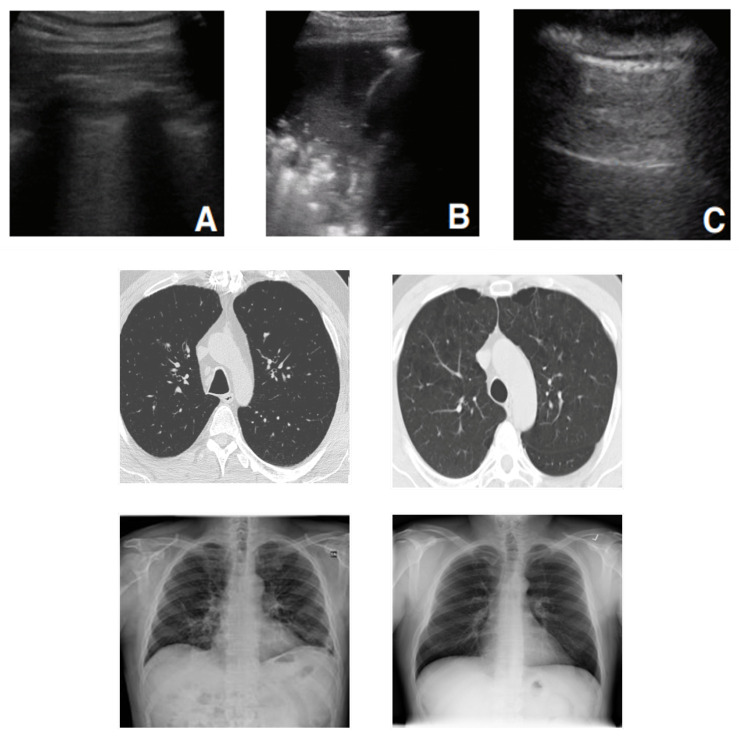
Visual-based modality types include: First row—Ultrasound (US) images representing (A) COVID-19-infected lung, (B) pneumonia, and (C) normal lung, respectively [81]. Second row—CT scan images of COVID-19 (left) and Non-COVID-19 (right) [85]. Third row—Chest X-ray (CXR) images of COVID-19 (left) and normal (right) [86].
